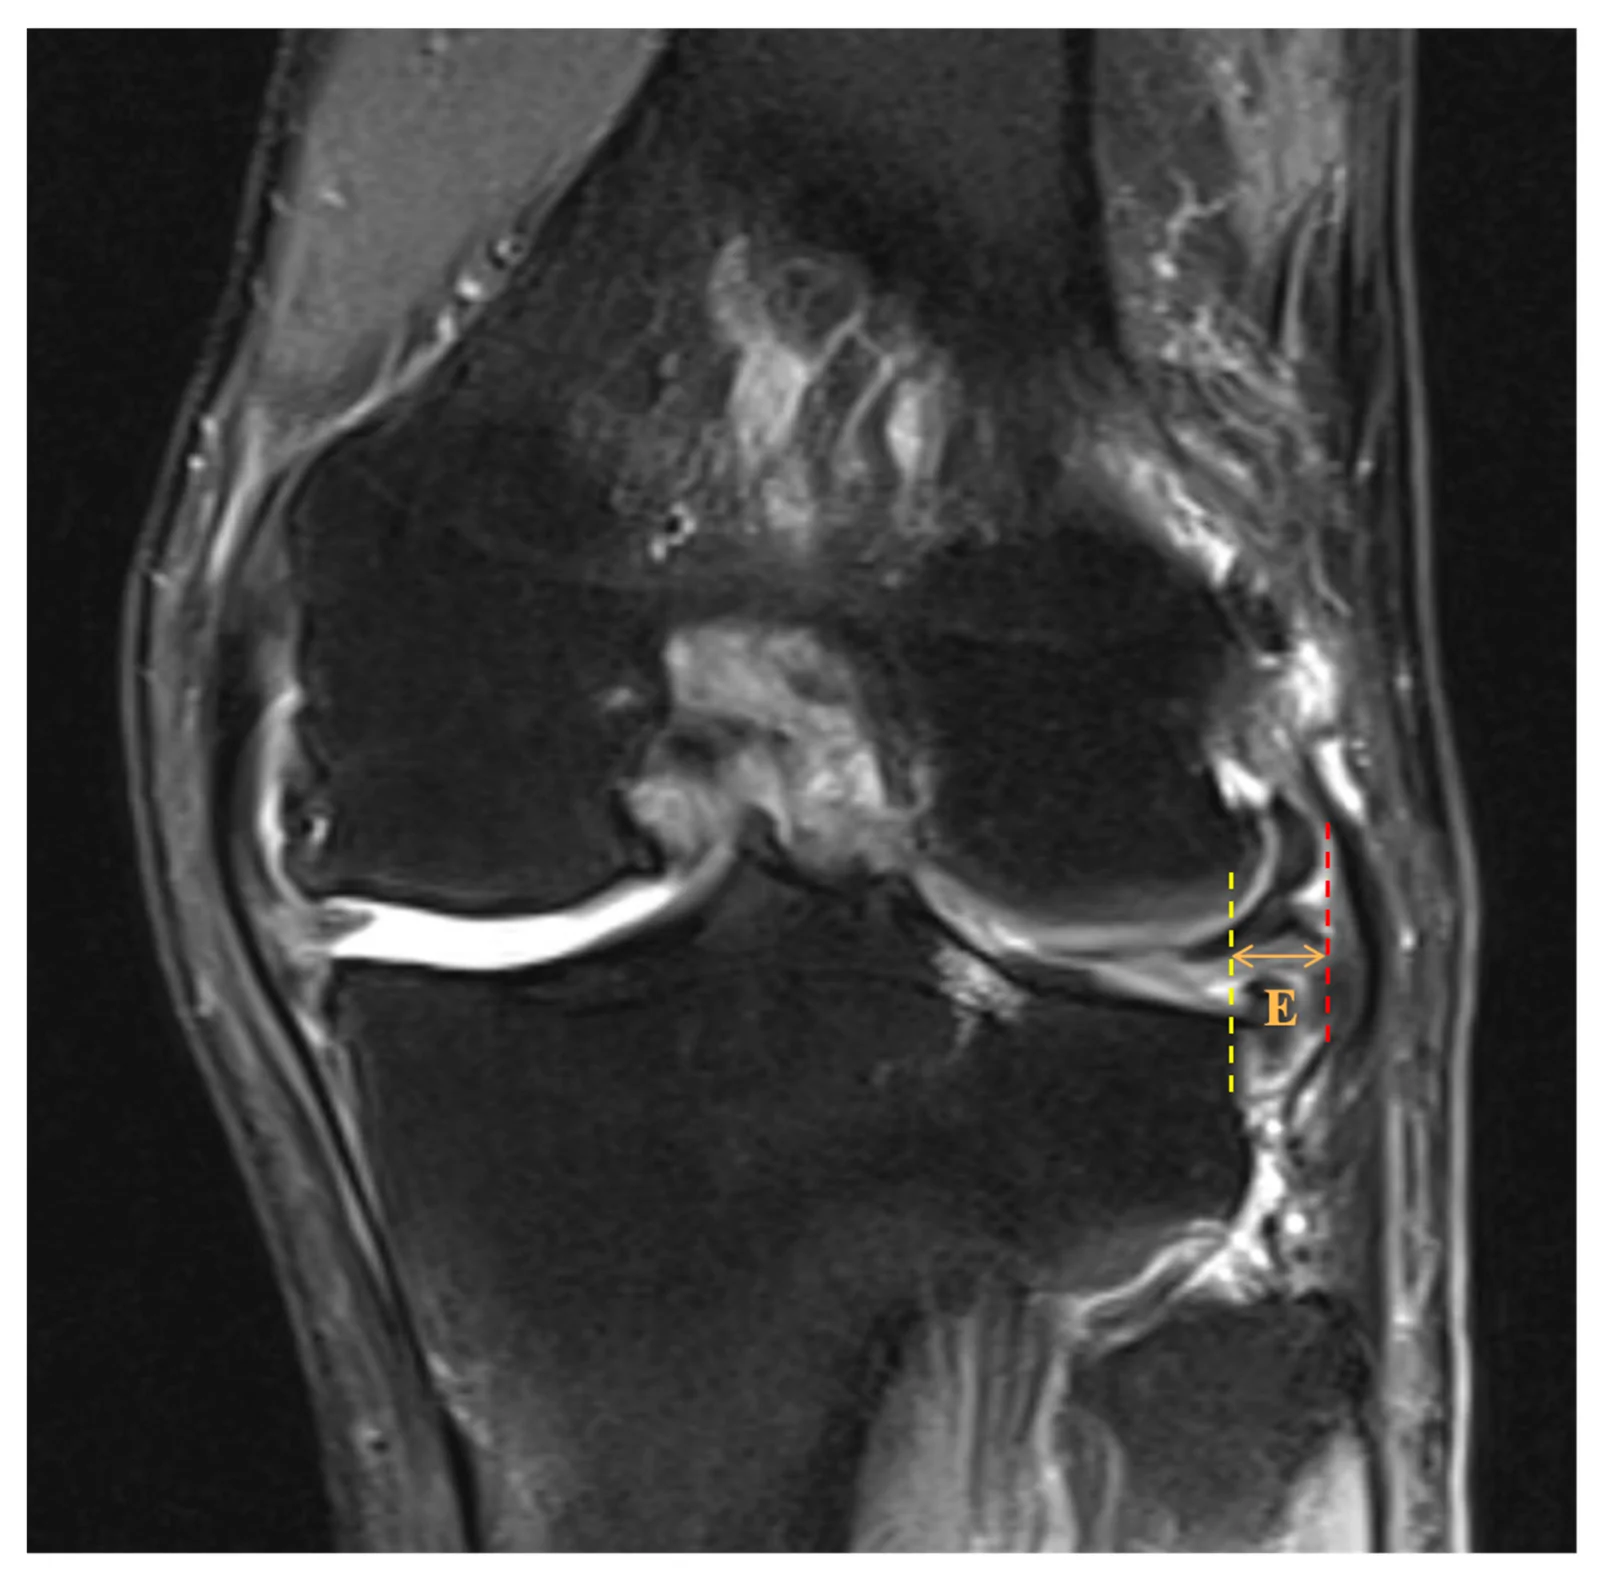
Representative coronal proton density fat-suppressed MRI demonstrating lateral meniscus extrusion (LME). LME was identified as displacement of the peripheral margin of the lateral meniscus beyond the lateral tibial plateau margin. Extrusion distance (E) was measured as the perpendicular distance between the lateral tibial plateau margin (yellow dashed line) and the peripheral margin of the lateral meniscus (red dashed line), representing the extent of lateral meniscus extrusion.
